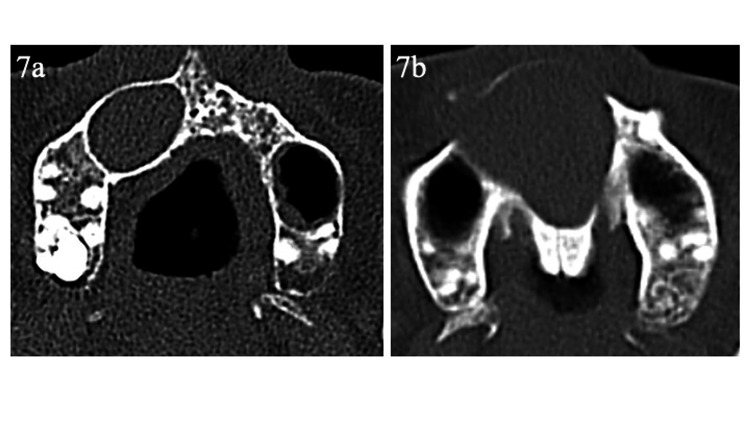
Representative computed tomography (CT) images of perilesional sclerotic signs 7a: Radicular cyst with a well-defined radiopaque sclerotic rim around the lesion, indicating a positive perilesional sclerotic sign; 7b: Radicular cyst without surrounding radiopaque changes, indicating a negative perilesional sclerotic sign.
